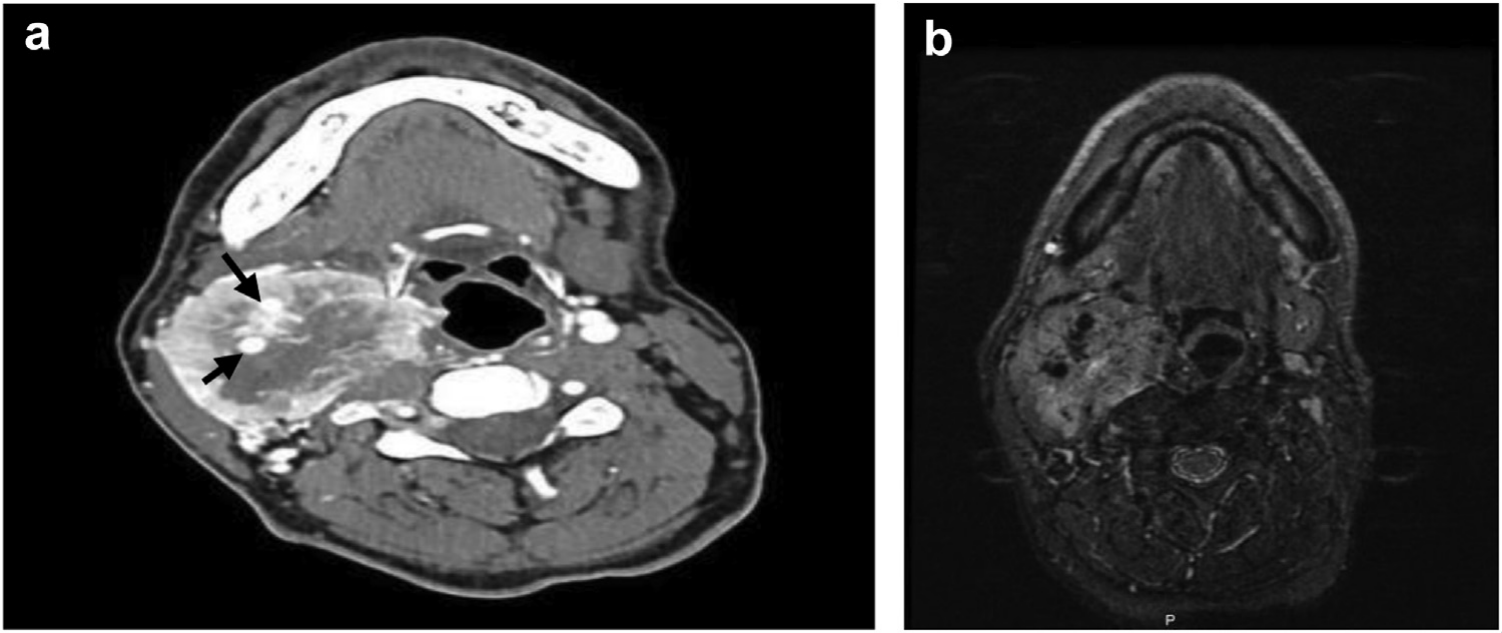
A, Computed tomography angiography (CTA) showing large carotid body tumor (CBT) with encasement of external and internal carotid arteries (ECA and ICA, respectively; *black arrows*). B, Magnetic resonance imaging (MRI) scan showing large carotid body tumor (CBT) encasing the ECA and ICA.
